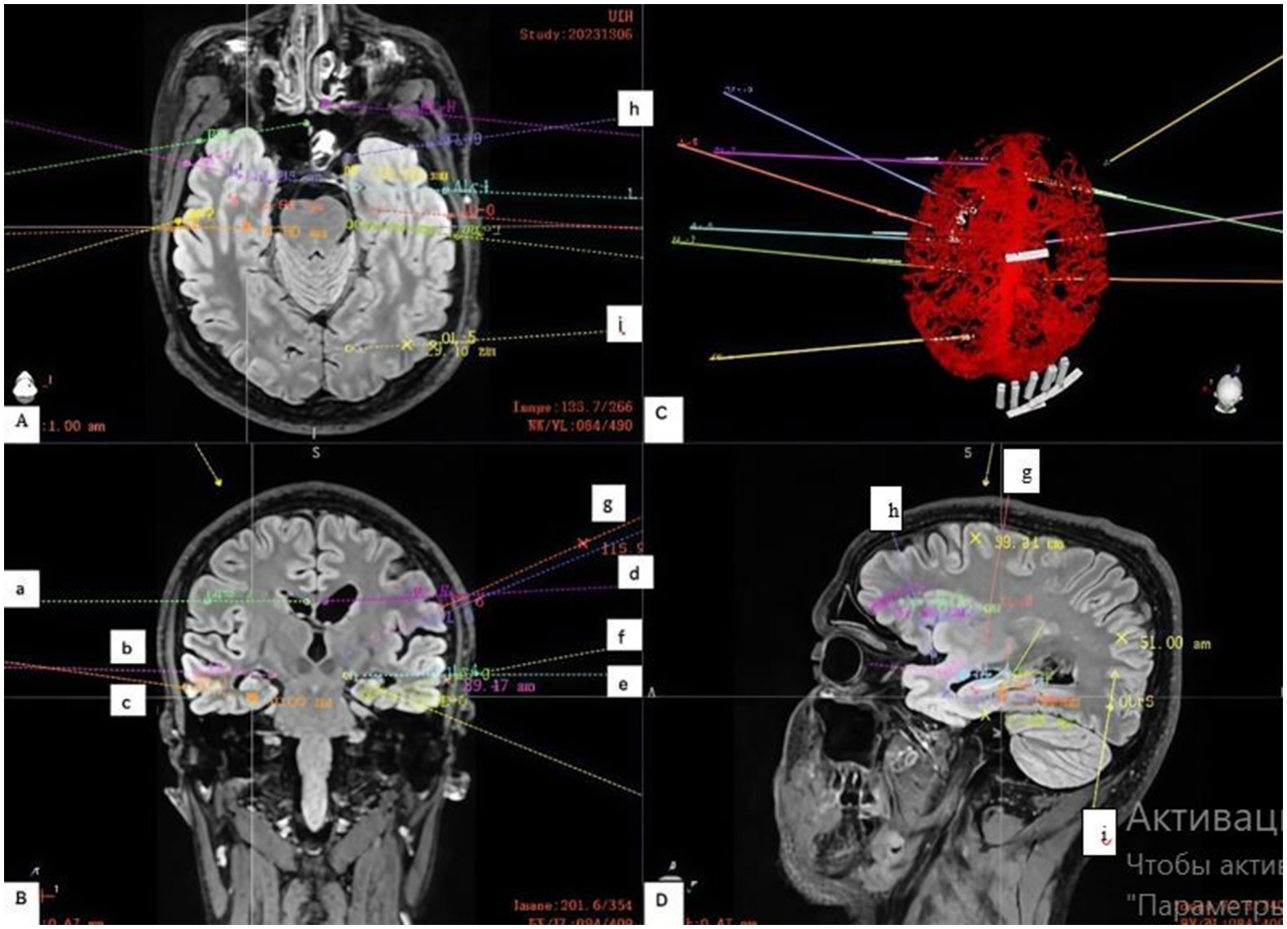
Stereoelectroencephalography (SEEG) Electrode Implantation Strategy. (A) Shows an axial view of the trajectories, targeting the: (h) left orbitofrontal cortex and (i) left occipital lobe. (B) Shows a coronal view with electrodes placed in key limbic structures: (a) right cingulate gyrus, (b) right amygdala, (c) right hippocampus; and (d) left cingulate gyrus, (e) left amygdala, (f) left hippocampus; and (g) left insular gyrus. (C) Demonstrates the planned electrode trajectories relative to the brain’s vascular structures, as confirmed by a contrast-enhanced computed tomography (CT) scan. (D) On the sagittal projection, the trajectory of the electrodes in the left hemisphere directed to the (h) orbitofrontal cortex, (g) insular gyrus; and (i) occipital lobe is more clearly visible.
